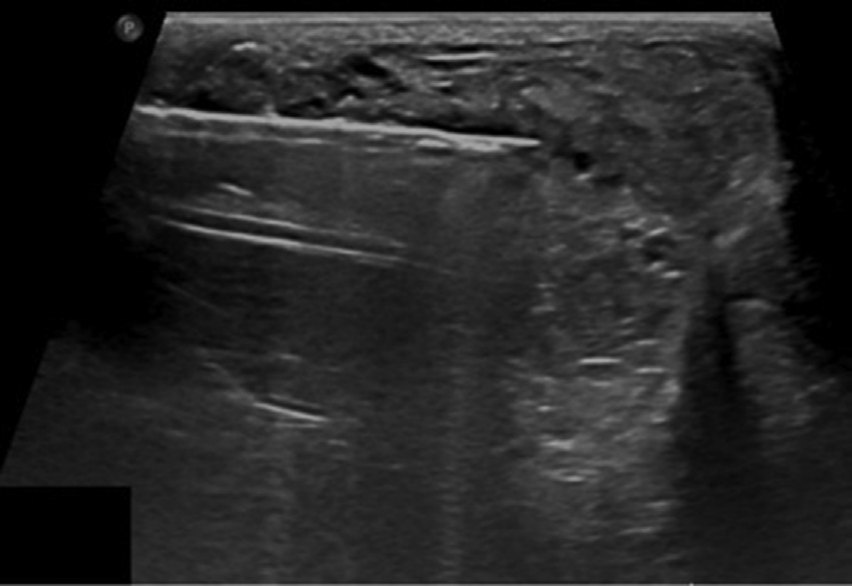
Ultrasound guided core biopsy with 14-gauge automated device of the right breast mass in the upper outer quadrant, 8 cm from the nipple.
